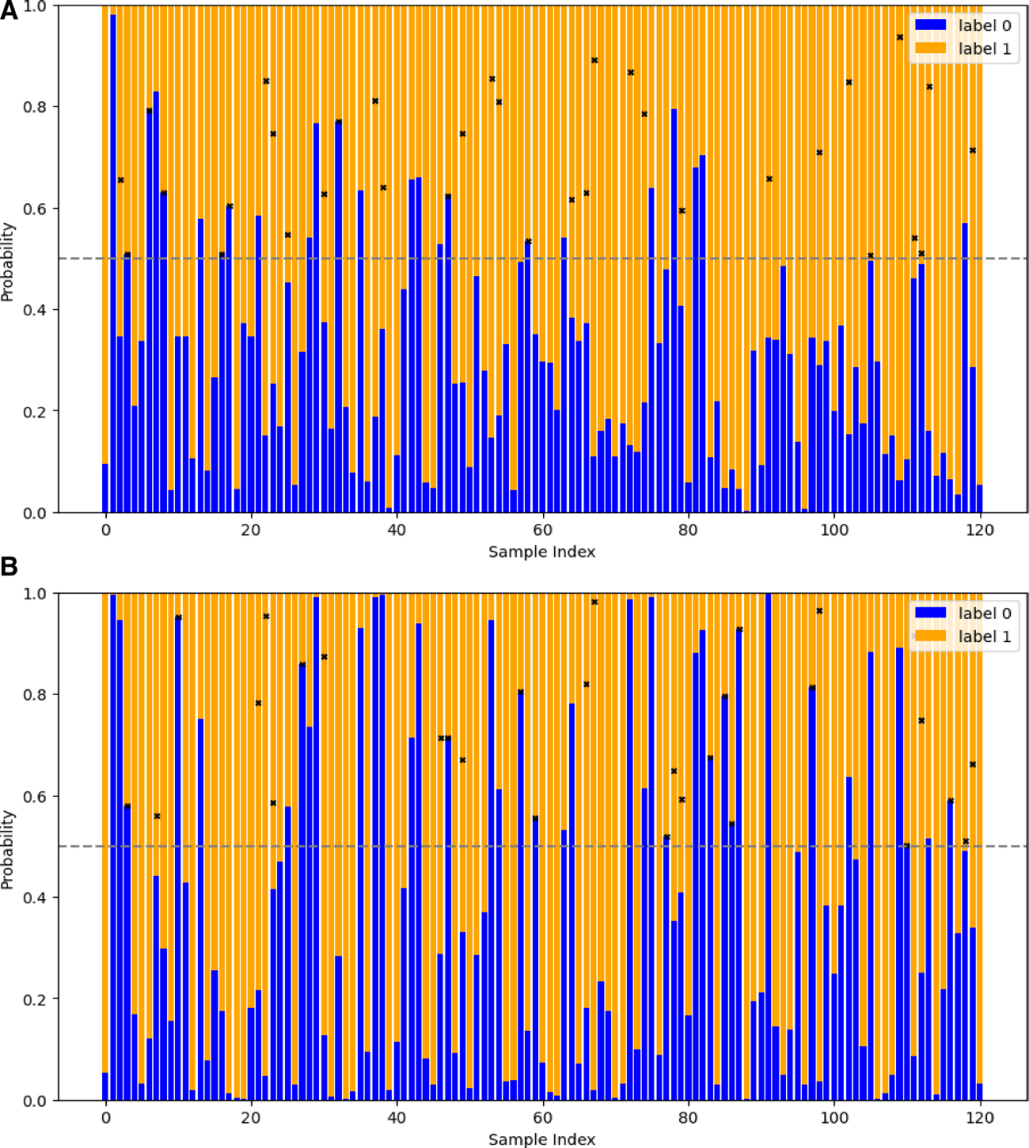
Prediction probability graph of test set. (A) prediction probability graph of the anteroposterior; (B) prediction probability graph of the lateral elbow radiographs. The horizontal coordinate is for each sample in the test set, the ordinate is the probability that the sample is predicted to be 0 or 1 by the logistic regression model, the dashed line is the threshold (0.5).
